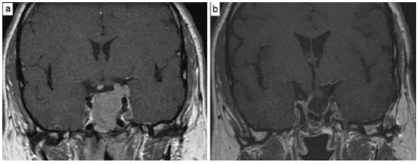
Magnetic resonance imaging (mri) in a 38-year-old man with adrenocorticotropic hormone–secreting adenoma. (a) Pretreatment image shows a large postoperative residual tumour. (b) Follow-up image 4 years after fractionated stereotactic radiotherapy shows complete response.
